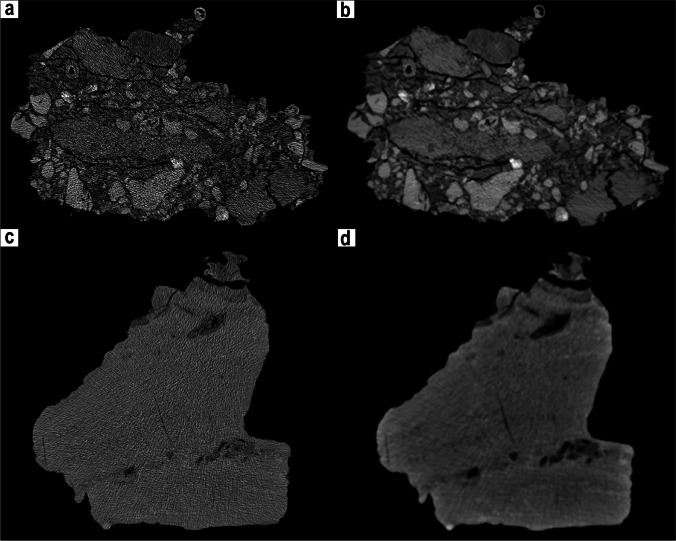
Raw data of the CT scans of MSF 89 (a, b) and MSF 62 (c, d) using different kernel values. The sets in a and c have higher values, so sharper scans, whereas b and d have lower kernel values, so more blurred. The use of these different presets in the scans deeply affects the possibility of discriminating between bone, matrix, and clasts
